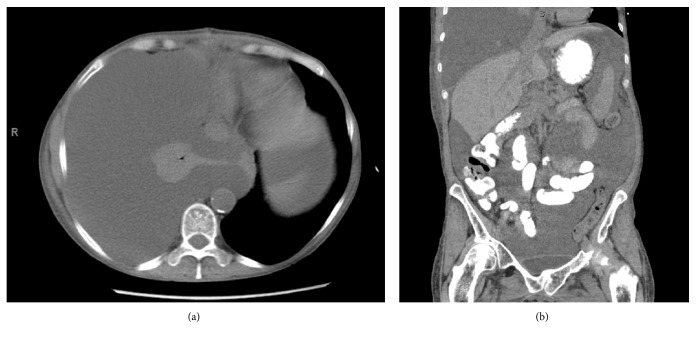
(a) Axial CT demonstrating large right pleural effusion with right lung atelectasis (b) Coronal CT scan demonstrating right chylothorax and chylous ascites.
